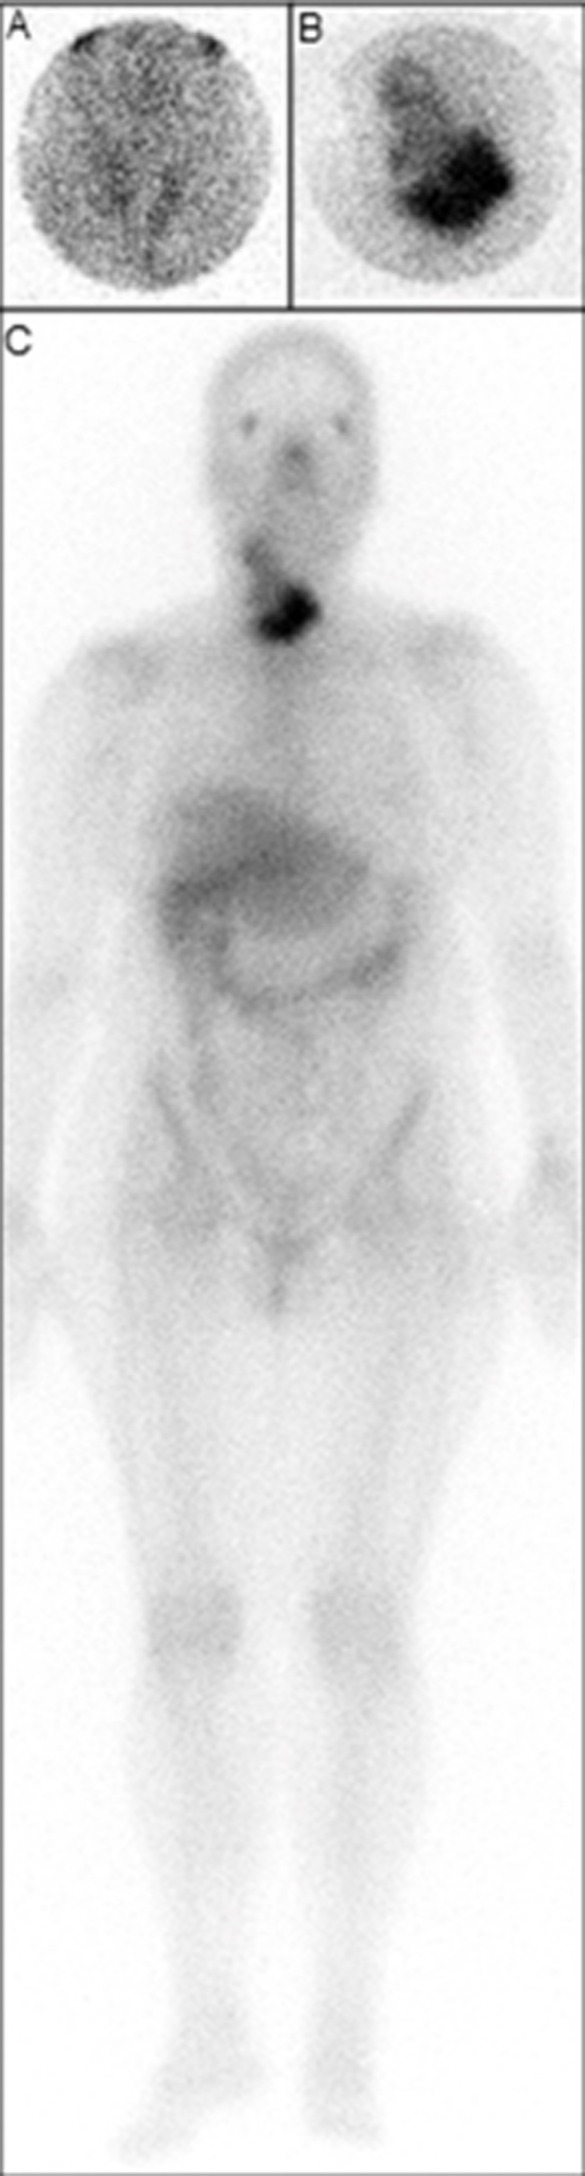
anterior-pinhole (magnified) images of the neck; A) technetium-99m scintigraphy, showing decreased thyroid uptake; B) gallium-67 scintigraphy, demonstrating marked accumulation in the thyroid lymphoma; C) whole-body gallium-67 scintigraphy
